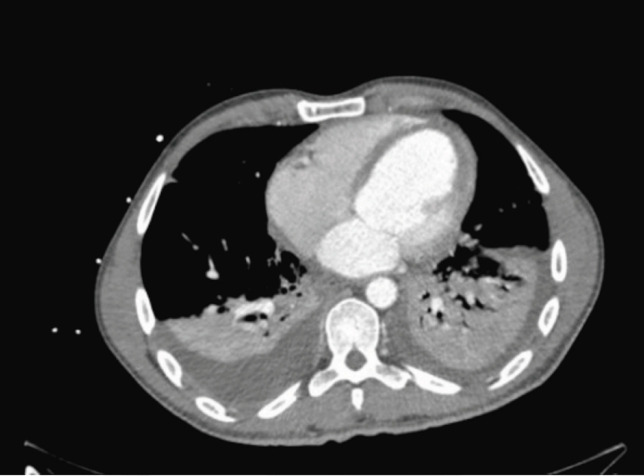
Anterior mediastinal haematoma on CT scan
